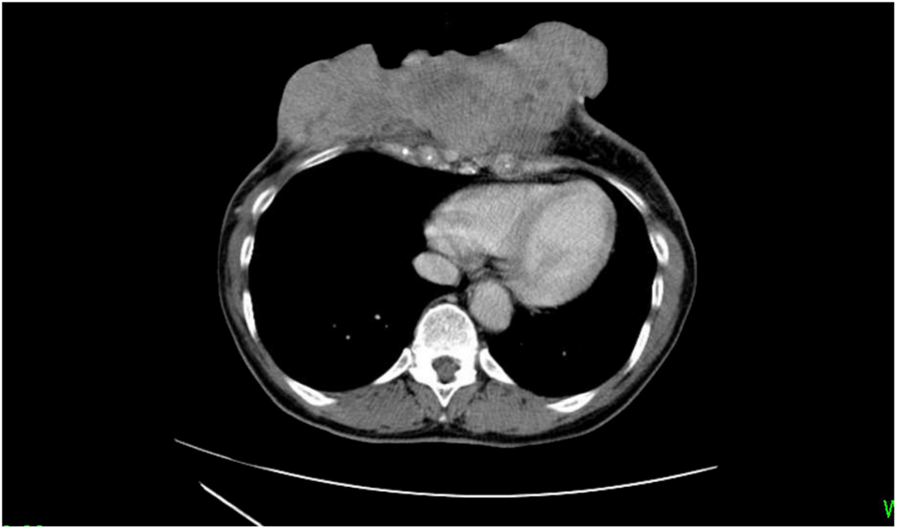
Computed tomography performed on the day of admission showing that the tumor had invaded the rib cage
